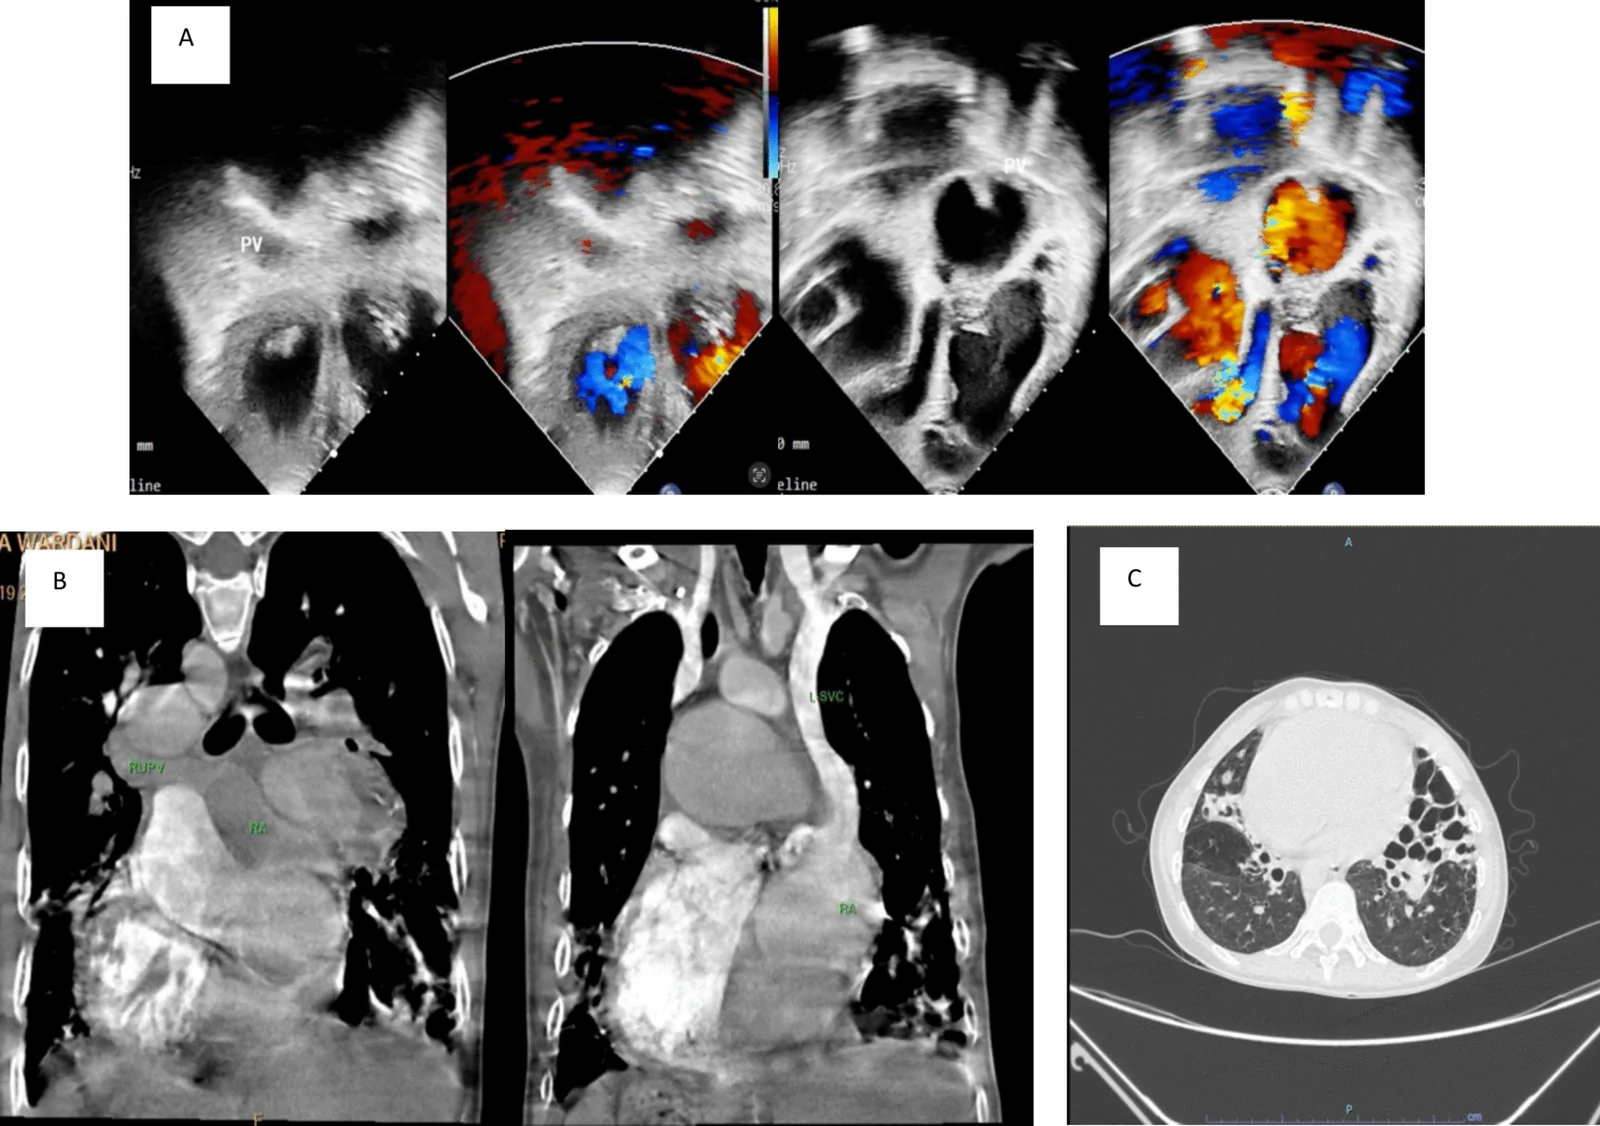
A Partial anomalous pulmonary venous drainage (PAPVD) shown in transthoracic echocardiography; B PAPVD shown in cardiac computed tomography reconstruction; C bronchiectasis in the middle land lower right lobe shown in high-resolution computed tomography
